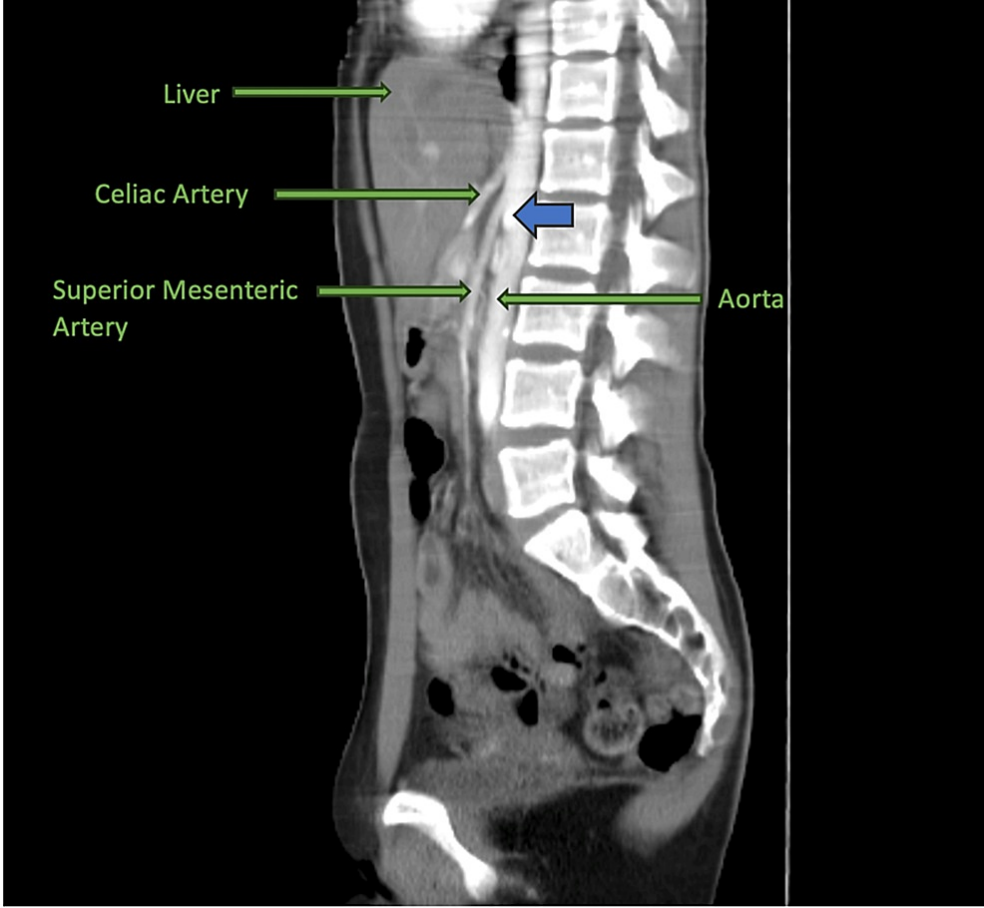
CT Abdomen Depicting an Aortomesenteric Angle of 19° Blue arrow pointing toward the angle between the aorta and superior mesenteric artery. The average normal aortomesenteric angle is 38°-56°.
